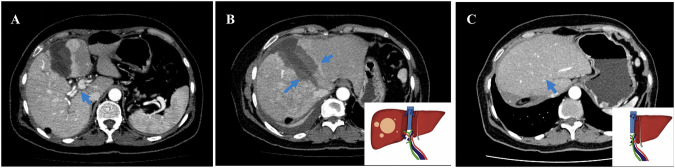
The procedure of ALPPS. A Portal vein ligation in the first stage. The maximum intensity projection of the CT image in the portal phase after the ALPPS stage 1 procedure shows no contrast filling in the right portal vein (arrow) as a result of right portal vein ligation. B Liver partition in the first stage. The axial enhanced CT image in arterial phase after ALPPS stage 1 procedure shows separation of the liver parenchyma (arrow) between the S4, S5 and S8. C Right hepatectomy in the second stage. The axial enhanced CT image after ALPPS stage 2 shows that the right liver lobe and gallbladder have been removed, and enlargement of the left lobe (arrow)
